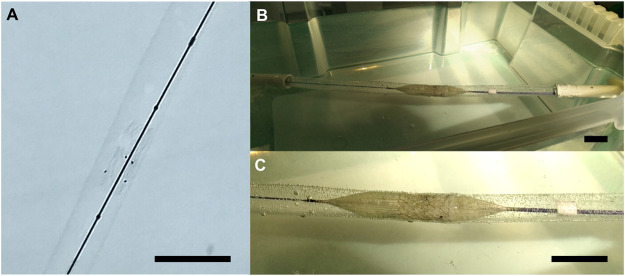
Representative images of the EVV in the balloon expansion transcatheter delivery. (A) EVV prior to balloon expansion, with four tantalum tubes sutured to facilitate X-ray visualization. (B) EVV upon balloon expansion. (C) Enlarged view of the EVV upon balloon expansion. Scale bar = 2.5 cm.
